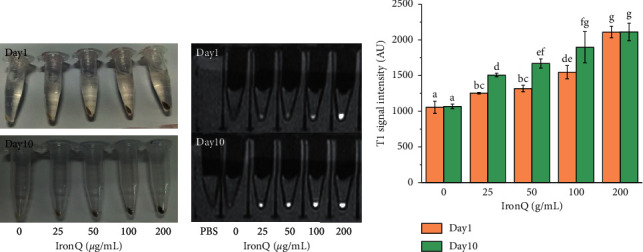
In vitro* MRI studies: (a) physical observations, (b) T1-weighted images from a sagittal view, and (c) a plot of the T1 signal intensity of PBMC pellets labeled with IronQ at various concentrations in microcentrifuge tubes with phosphate buffer saline (PBS) on day 1 and day 10 of the incubation period. Different lowercase letters indicate significant difference according to the ANOVA analysis followed by Tukey's test (*n* = 3; *p* < 0.05).
